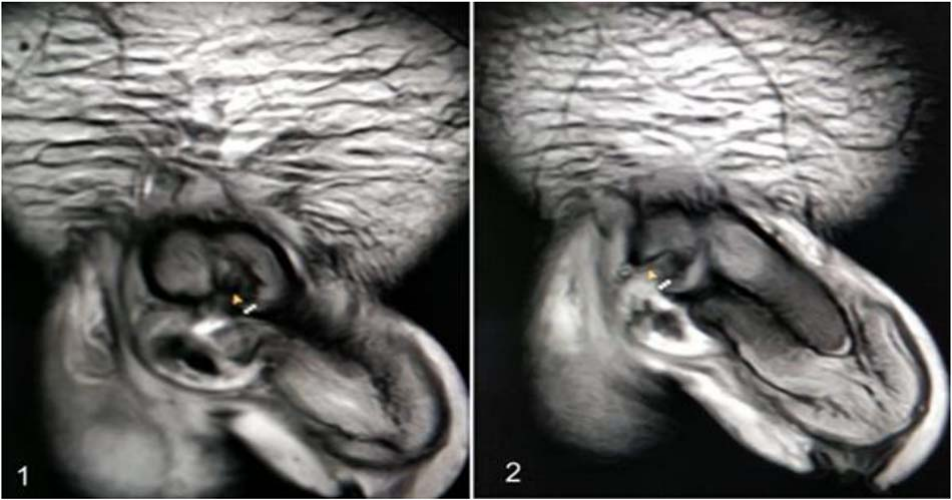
An MRI with (1) left corporeal tear on the left side with surrounding hematoma and (2) right corporeal tear with hematoma and disruption of urethra.
